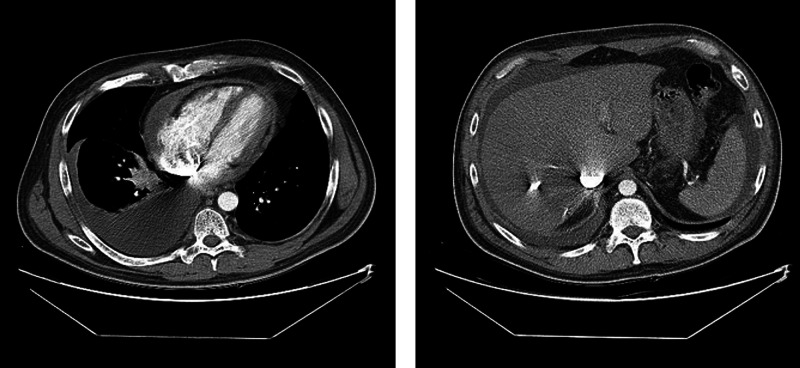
CTPA showing right pleural effusion with adjacent atelectasis, pericardial effusion, and mild ascites. CTPA: computed tomography pulmonary angiography.
